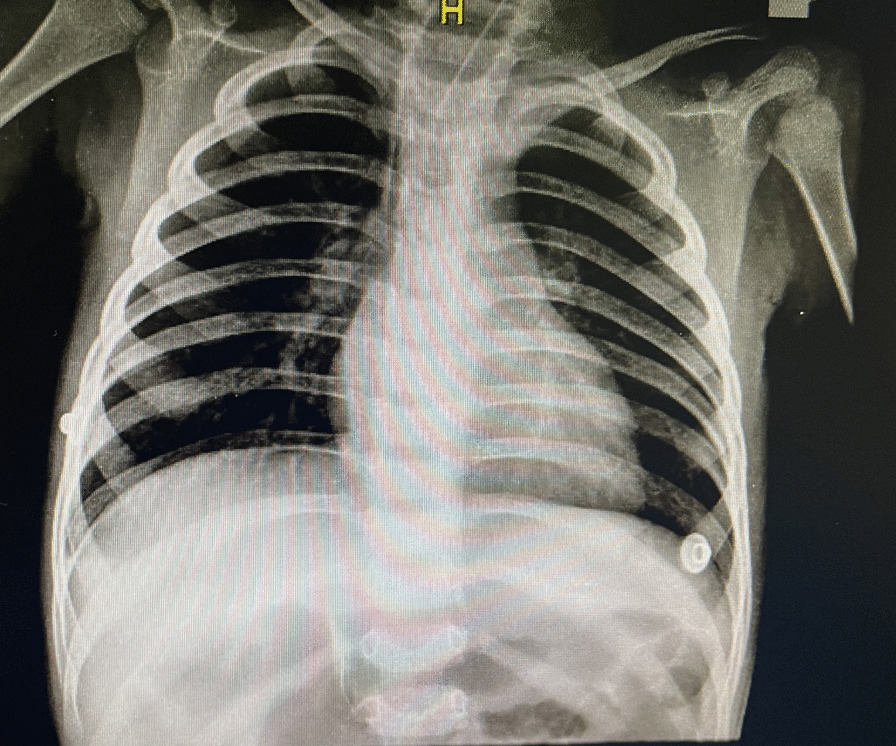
A chest radiograph showing lung involvement in the right peripheral area
